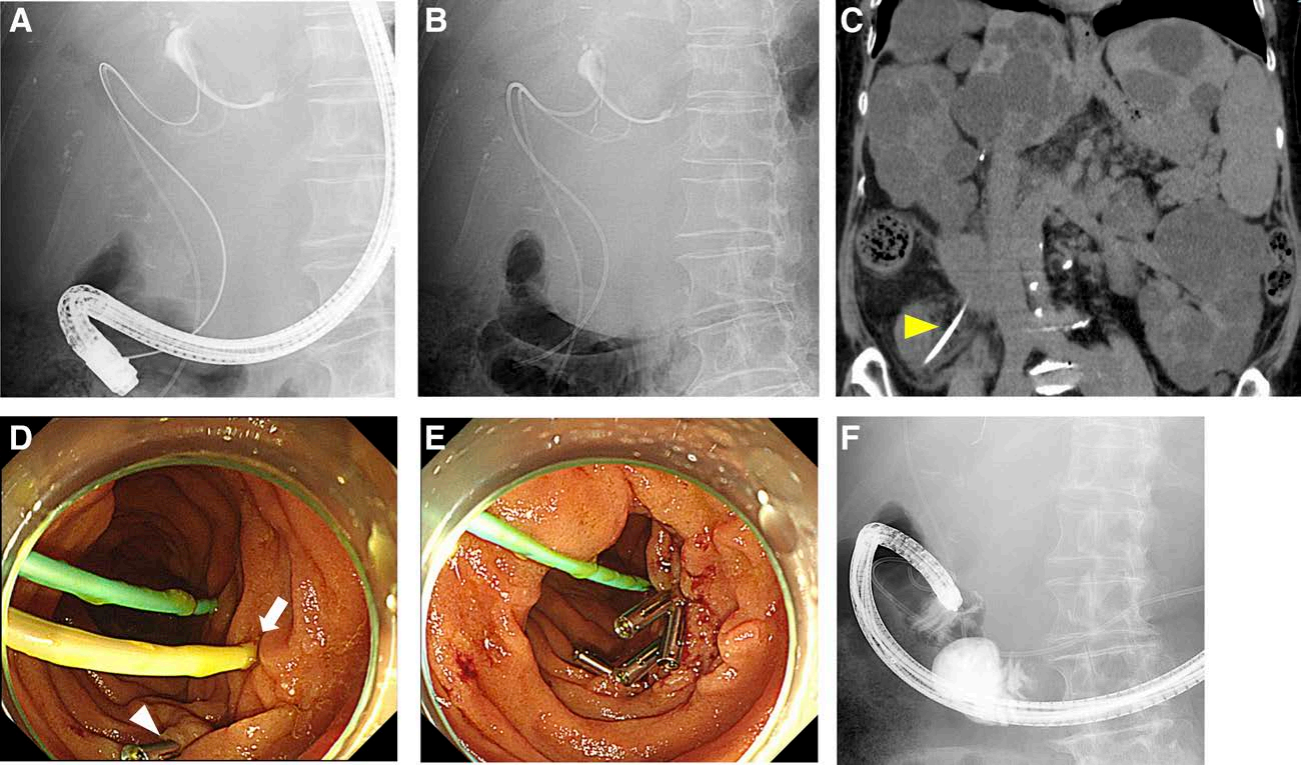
(A): The patient underwent ERCP due to stenosis of the intrahepatic bile duct caused by multiple liver cysts. (B): The patient underwent biliary drainage using a 5 Fr ENBD tube cut to 28 cm for B5 and a 6 Fr ENBD tube cut to 25 cm for B4. (C): CT revealed distal migration of the PS for B4 and duodenal perforation (yellow arrowhead). (D): The distal tip of the biliary stent penetrated the duodenal wall (white arrow). This was confirmed during endoscopy after marking the perforation site with hemoclips (white arrowhead). (E): The ENBD tube was removed using a forceps, and the perforated duodenal wall was closed using hemoclips. (F): Contrast injection after closure of perforation site showed no residual leakage. CT = computed tomography, ENBD = endoscopic nasal biliary drainage, ERCP = endoscopic retrograde cholangiopancreatography, PS = plastic stent.
